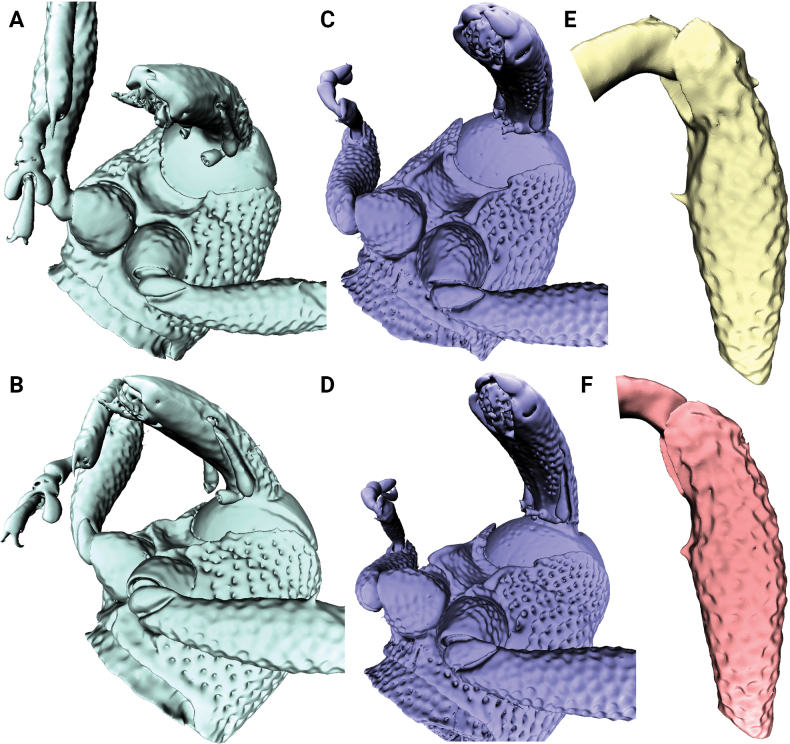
X-ray microtomography generated 3D models of *Aphanerostethus*A, B*A.armatus* sp. nov. (JHLHY_DAR_078) shallow prosternal canal C, D*A.magnus* sp. nov. (JHLHY_DAR_029) deep prosternal canal E*A.armatus* (JHLHY_DAR_078) fore-femur showing elongate, thorn-like ventral tooth F*A.japonicus* sp. nov. (JHLHY_DAR_051) fore-femur showing blunt, obtuse ventral tooth.
